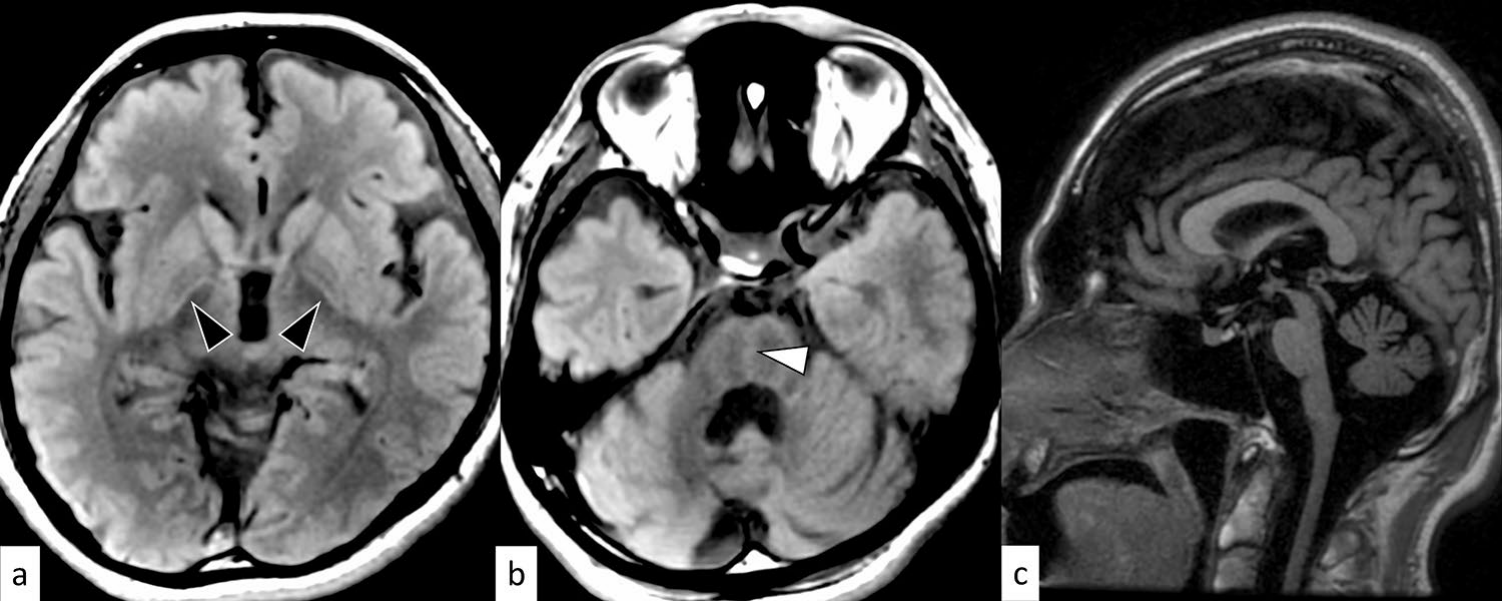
A 41-year-old man with SCA3/MJD. Proton-weighted axial images show hyperintensity bands in the bilateral medial segments of the globi pallidi (a, black arrowheads) and an incomplete hot cross bun sign (b, white arrowhead). T1-weighted sagittal image shows an atrophy of the brainstem and cerebellum (c)
